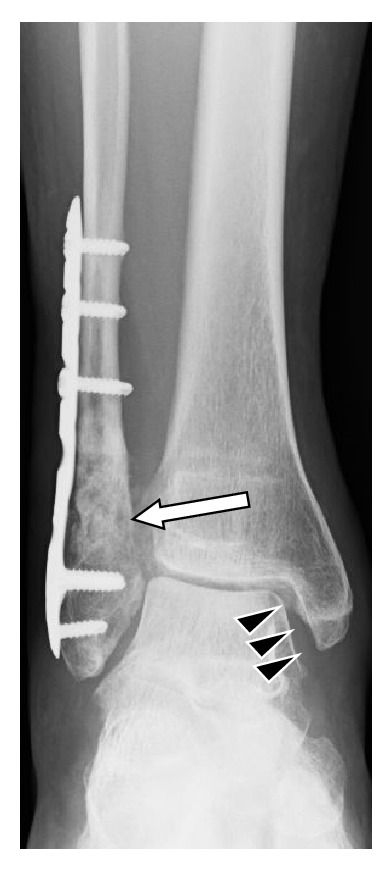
Radiograph 5 months after the 2nd operation. All abnormalities displayed in Figure 3 have been reconstructed to normal with the treatment that has been performed. Concerning fibula, grafted bone was not absorbed and alignment was not changed after second operation (white arrow). This properly aligned fibula, tightened ankle fork, and restored valgus tilt of the talus maintained medial clear space properly (black triangle).
